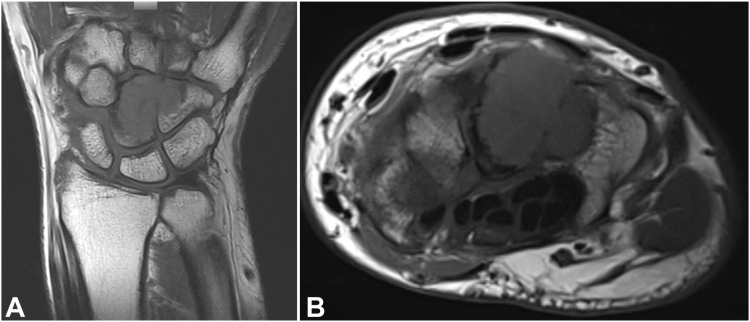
A, B Magnetic resonance imaging T1-weighted coronal and axial sections showing an isointense expansile, destructive lesion originating in the capitate with extension into the hamate.
